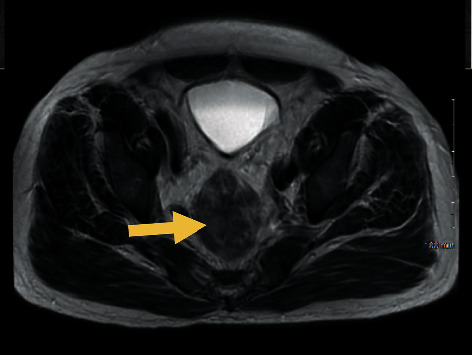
Axial image of MRI pelvis showing a nonspecific rectal mass (arrow).
